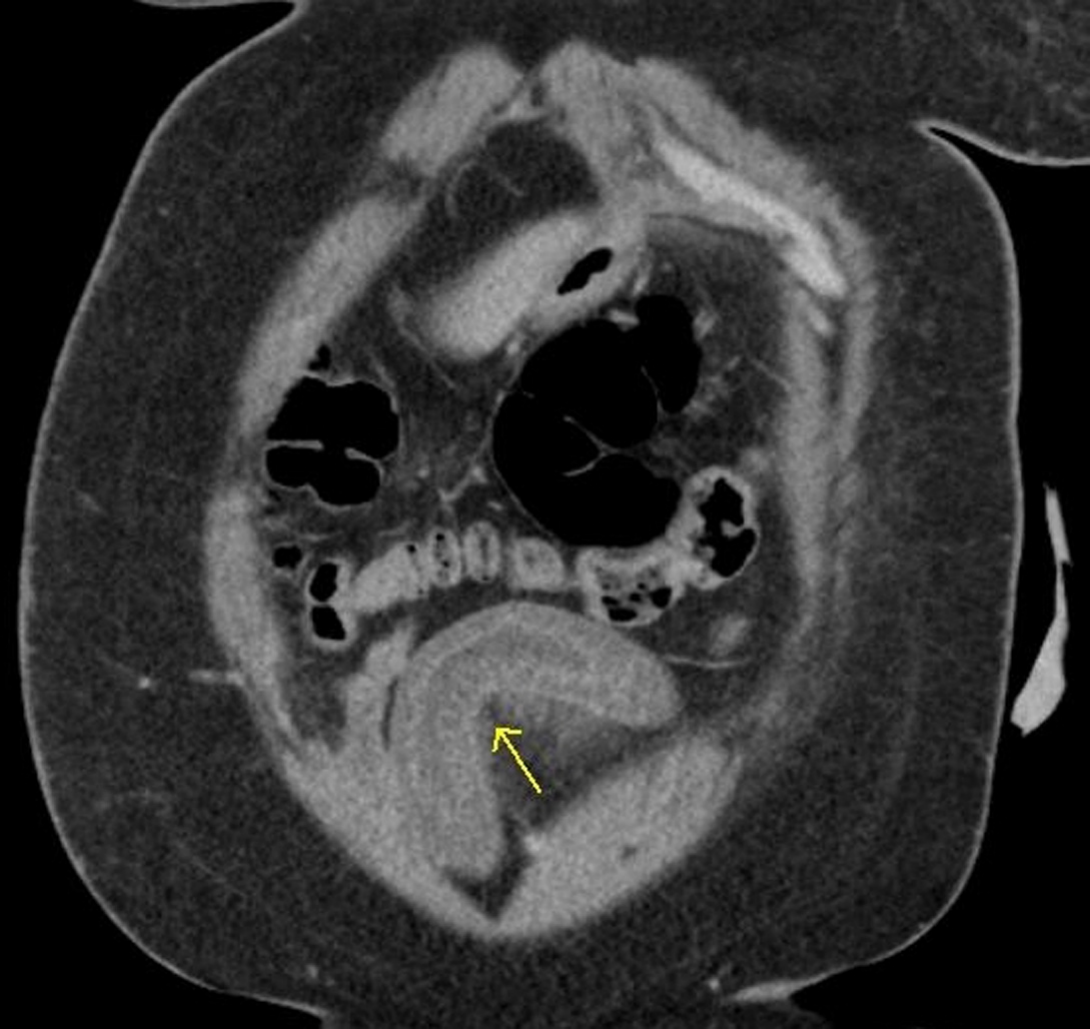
Coronal contrast‐enhanced CT image demonstrated thickening of the bowel wall with submucosal edema and all layers of the bowel delineated. (Yellow arrows).
